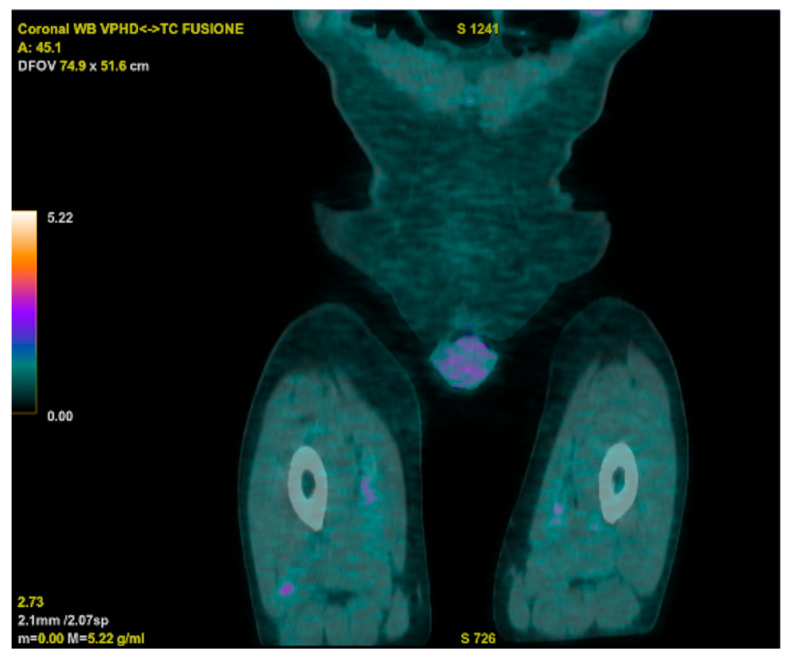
18 FDG PET-CT showing two hypercaptant lesions in the patient’s right thigh.
